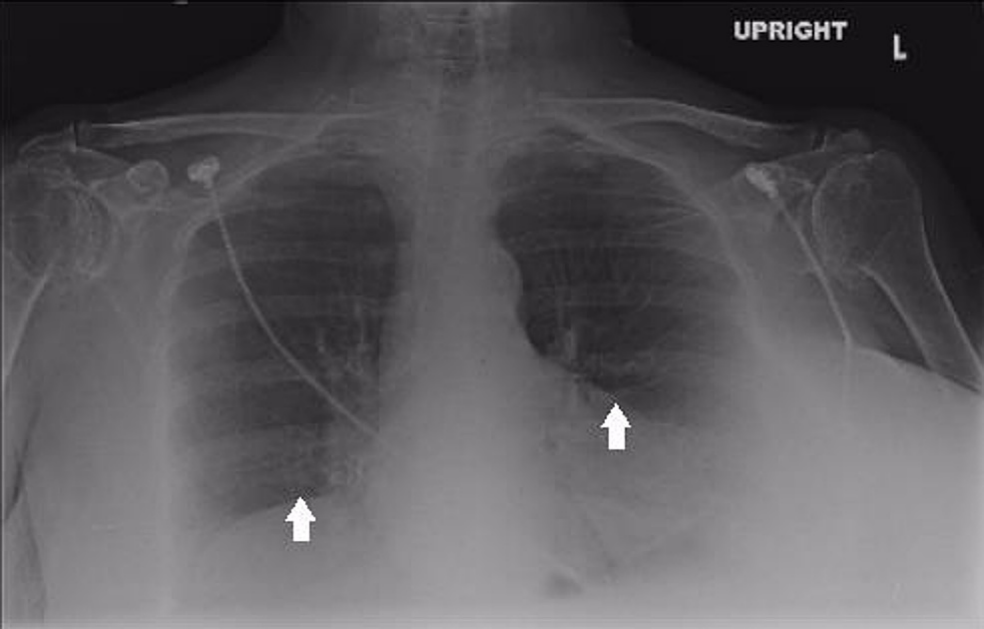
The chest X-ray shows shallow lung volumes with minor bibasilar atelectasis.
